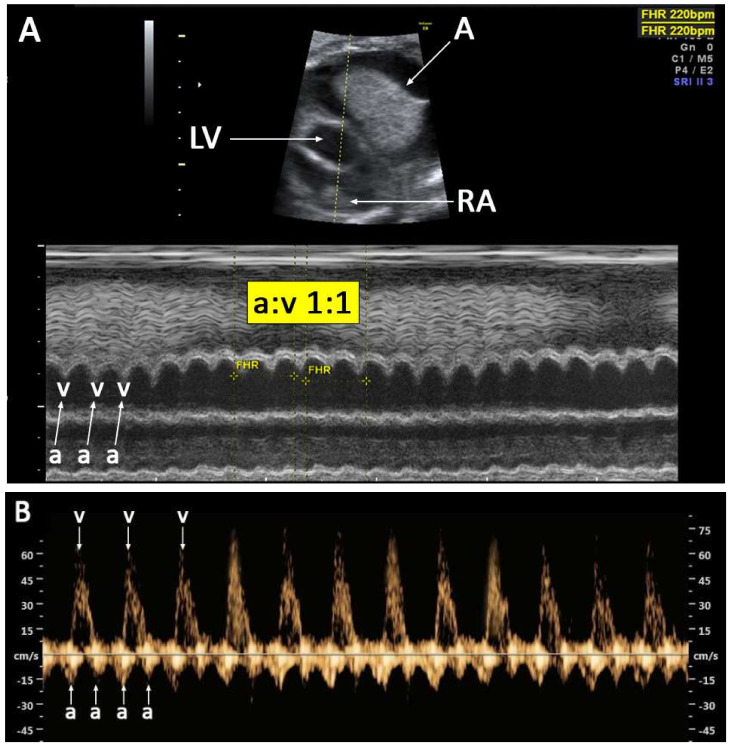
(A) M-mode shows supraventricular tachycardia; atrial rate (a): ventricular rate (v) (A): ascites; LA: left atrium; RA: right atrium); (B) cardiac Doppler waveforms at left inflow and outflow shows atrial flutter; atrial rate (a): ventricular rate (v) 2:1 (440:226).
